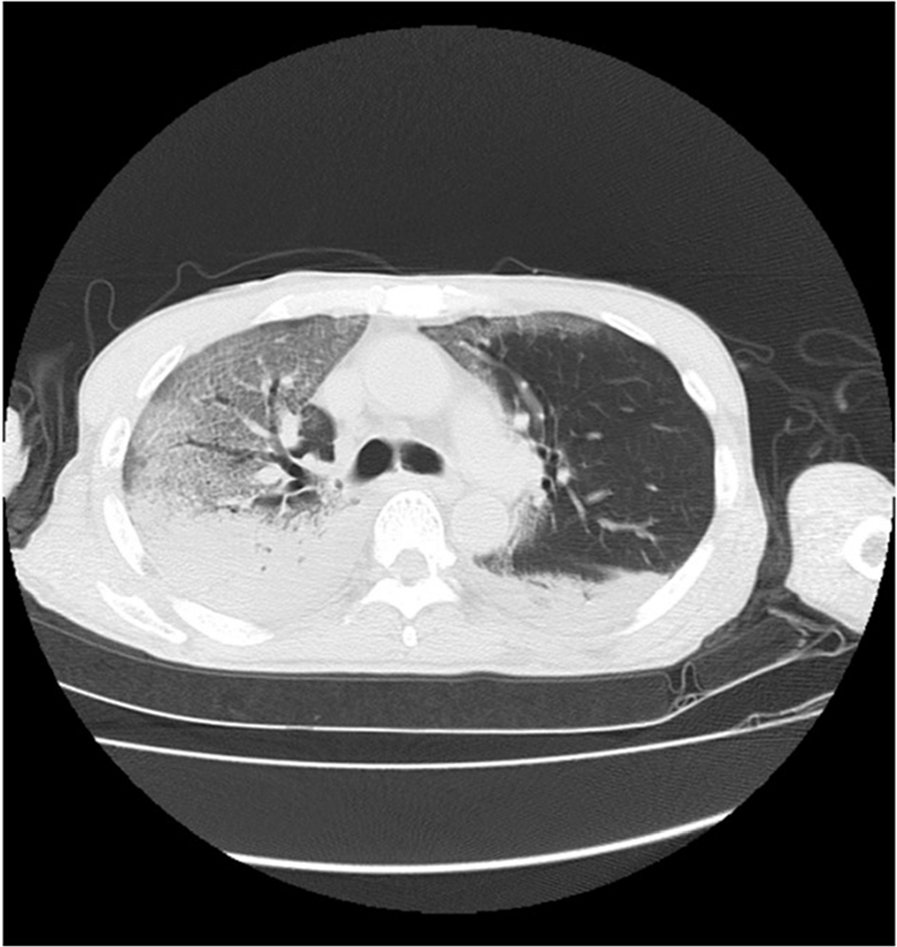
Computed tomography of the chest demonstrating bilateral patchy ground-glass opacities with interlobular septal thickening consistent with the crazy paving pattern found in patients with coronavirus disease 2019
